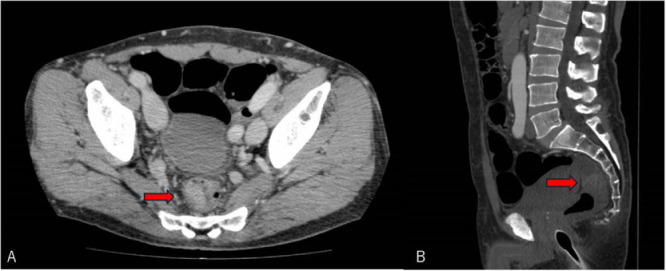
Preoperative contrast-enhanced CT images demonstrating the primary rectal tumor. (A) Axial contrast-enhanced CT showing a contrast-enhancing mass in the middle rectum (arrow). (B) Sagittal contrast-enhanced CT showing the same rectal tumor (arrow).
